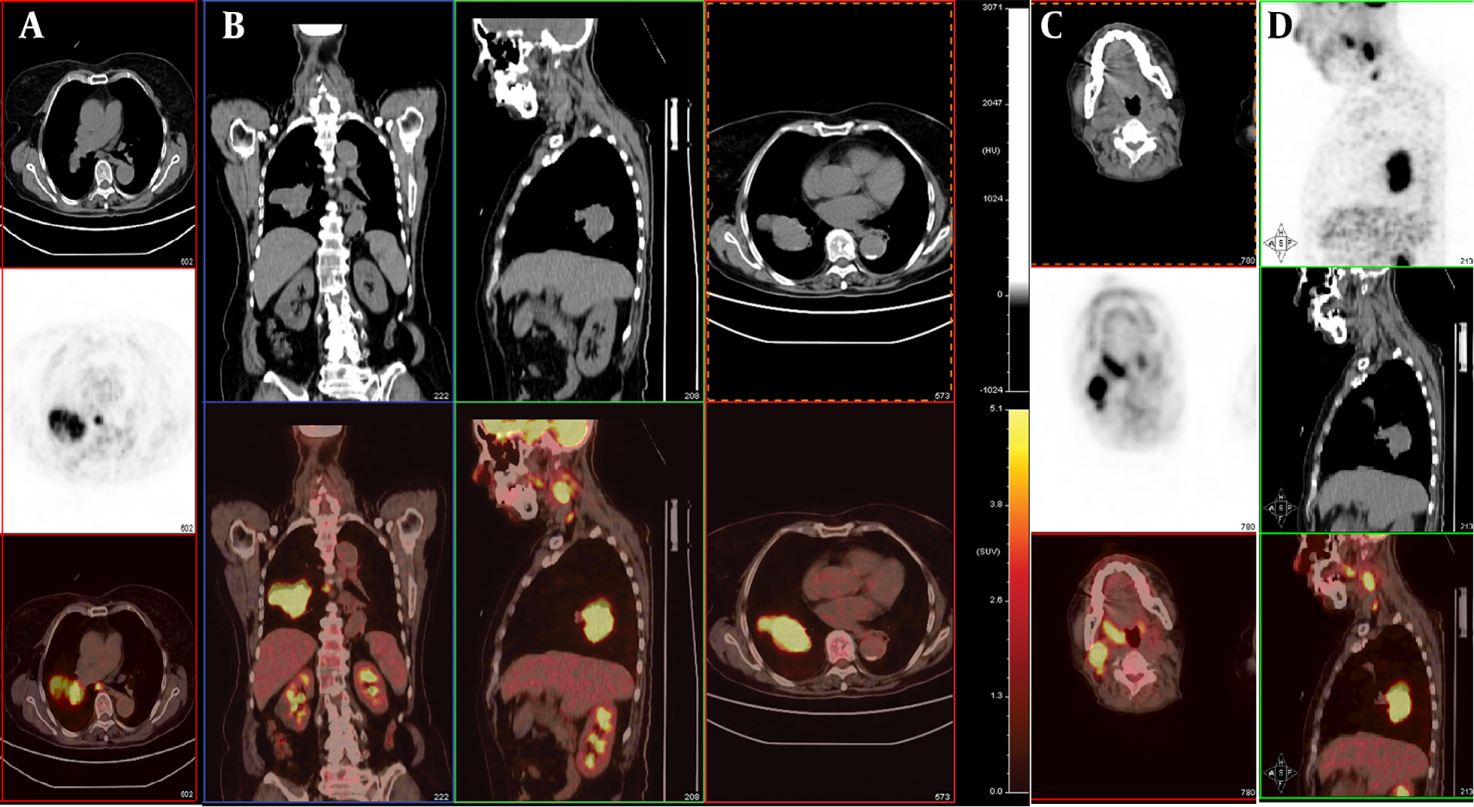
A and B, PET/CT images reveal multiple regions of increased FDG activity in the right lung (SUVmax=11.6) and mediastinal lymph nodes (SUVmax=7.9). C, The images show increased FDG uptake in the right oropharynx (SUVmax=7.3) and multiple intensely 18F-FDG-avid right submaxillary lymph nodes (SUVmax=13.6). D, The image shows increased FDG uptake in the oropharynx
